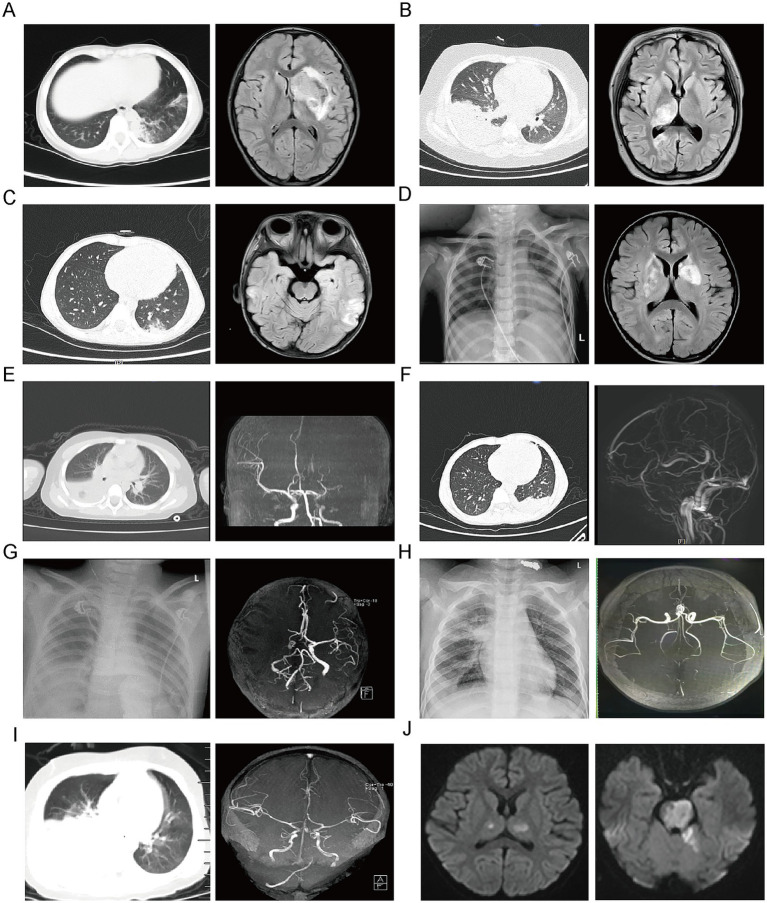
Radiologic manifestations in nine children with cerebral infarction (CI) complicating *Mycoplasma pneumoniae* pneumonia. (A) Patient 1: Chest CT showing left-sided pneumonia (left); brain MRI demonstrating abnormal signal intensity in the left frontoparietal cortex with involvement of the lateral ventricle (right). (B) Patient 2: Chest CT revealing large consolidation in the right lower lobe (left); brain MRI showing multiple irregular patchy lesions involving the right thalamus, right occipital lobe, and bilateral cerebellar hemispheres (right). (C) Patient 3: Chest CT showing right-sided pneumonia (left); brain MRI demonstrating abnormal signal intensity in the left temporo-occipital lobe (right). (D) Patient 4: Chest CT demonstrating bilateral pneumonia (left); brain MRI revealing multifocal abnormal signal intensities in the bilateral basal ganglia and cerebral peduncles (right). (E) Patient 5: Chest CT showing left-sided pneumonia with pleural effusion (left); magnetic resonance angiography (MRA) demonstrating non-visualization of the left anterior cerebral artery and middle cerebral artery and their branches (right). (F) Patient 6: Chest CT demonstrating consolidation in the left lower lobe (left); magnetic resonance venography showing non-visualization of the superior sagittal sinus and adjacent superficial cortical veins (right). (G) Patient 7: Chest radiograph showing right-sided pneumonia with pleural effusion (left); MRA demonstrating occlusion of the right middle cerebral artery (right). (H) Patient 8: Chest radiograph showing right upper- and lower-lobe pneumonia (left); MRA demonstrating absence of flow-related signal in the basilar artery and the P1 segments of the bilateral posterior cerebral arteries (right). (I) Patient 9: Chest CT showing right upper- and lower-lobe pneumonia (left); MRA demonstrating poor visualization of the distal basilar artery and non-visualization of the P1 segments of the bilateral posterior cerebral arteries (right). (J) Patient 9: Diffusion-weighted imaging demonstrating abnormal signal intensities in the thalamus (left), brainstem, and cerebellum (right).
